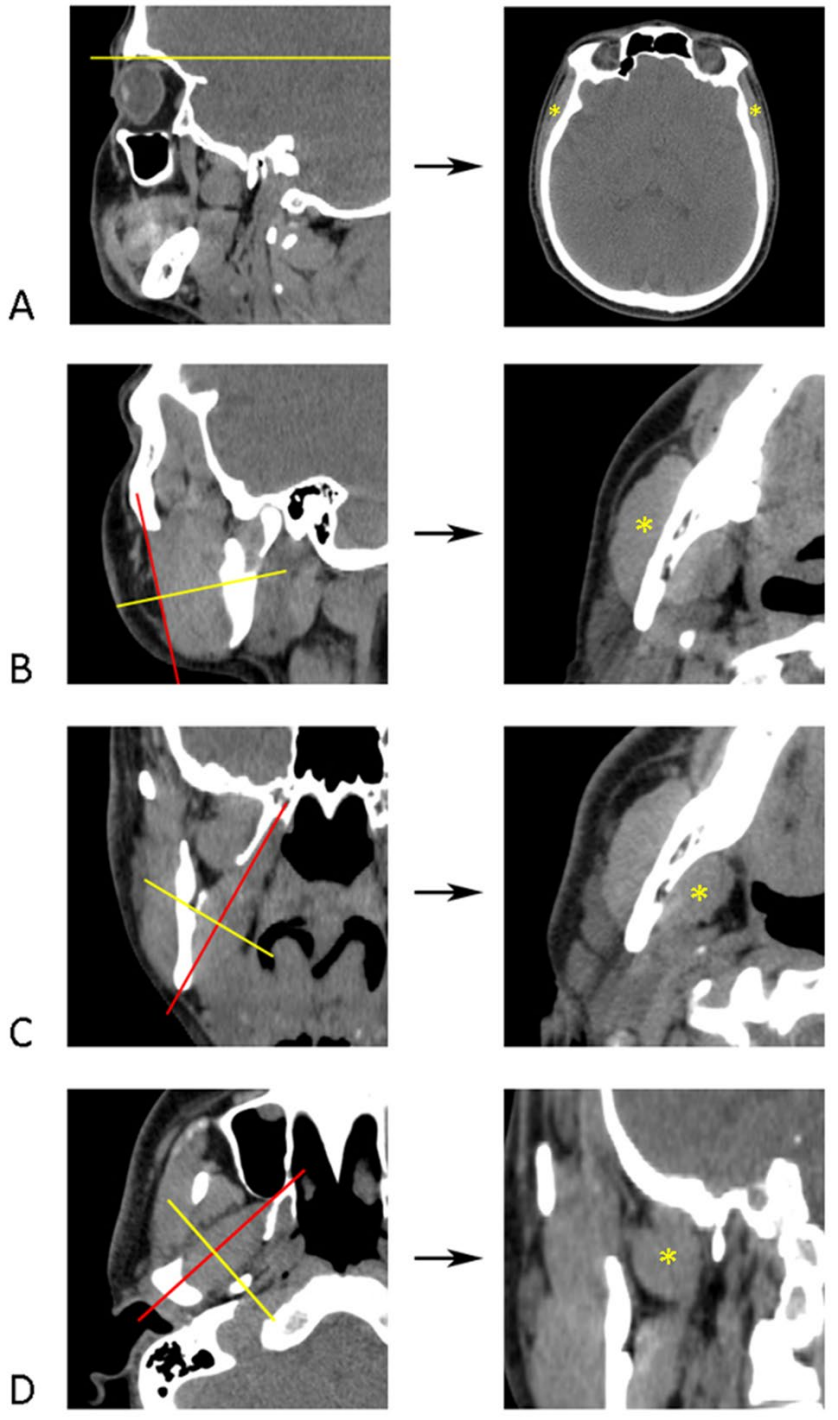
Selection of reference CT images of masticatory muscles for CSA measurement. (A) Axial CT image at the level of the superior orbital margin (yellow line) used as a reference image for the temporal muscle measurement. (B) The reference CT slice at the level of the mandibular foramen (yellow line) for the masseter measurement was selected perpendicular to the muscle long axis (red line). (C) For the medial pterygoid, the selected CT image at the level of the mandibular foramen (yellow line) was perpendicular to the muscle’s long axis (red line connects the inferior margin of the mandible and the top of the pterygoid fossa). (D) The reference CT slice for the lateral pterygoid measurement was selected at the middle of the muscle (yellow line) perpendicular to the muscle’s long axis (red line).
